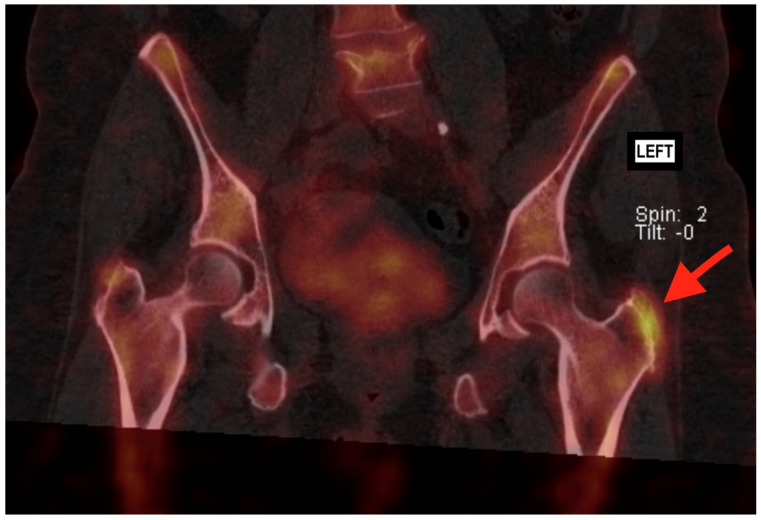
SPECT-CT (Tc-99m bone scintigraphy) imaging of a female patient with a clear hotspot on the left hip (red arrow). Indicating high bone activity in relation to spur formation.
